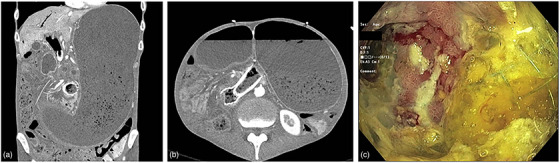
Computed tomography scan demonstrating severe gastric distention and prior biliary and enteric stents in situ (a and b). Endoscopic image of the enteric stents with tissue ingrowth (c).
